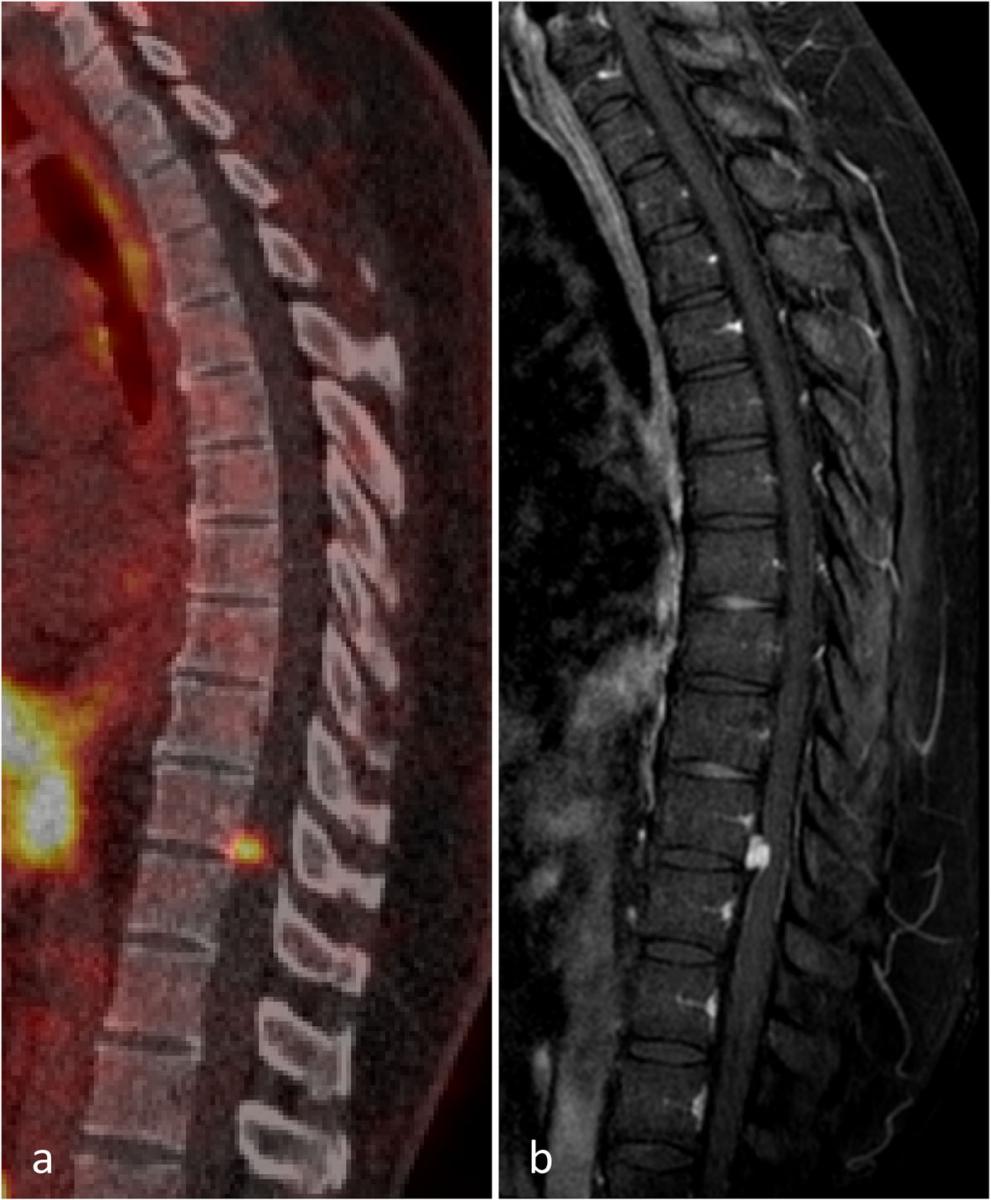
Images of patient 1. a Uptake dorsal of the tenth thoracic vertebra suspicious for leptomeningeal/drop metastasis on ^68^Ga-PSMA PET/CT. b Confirmation of metastasis on MRI
